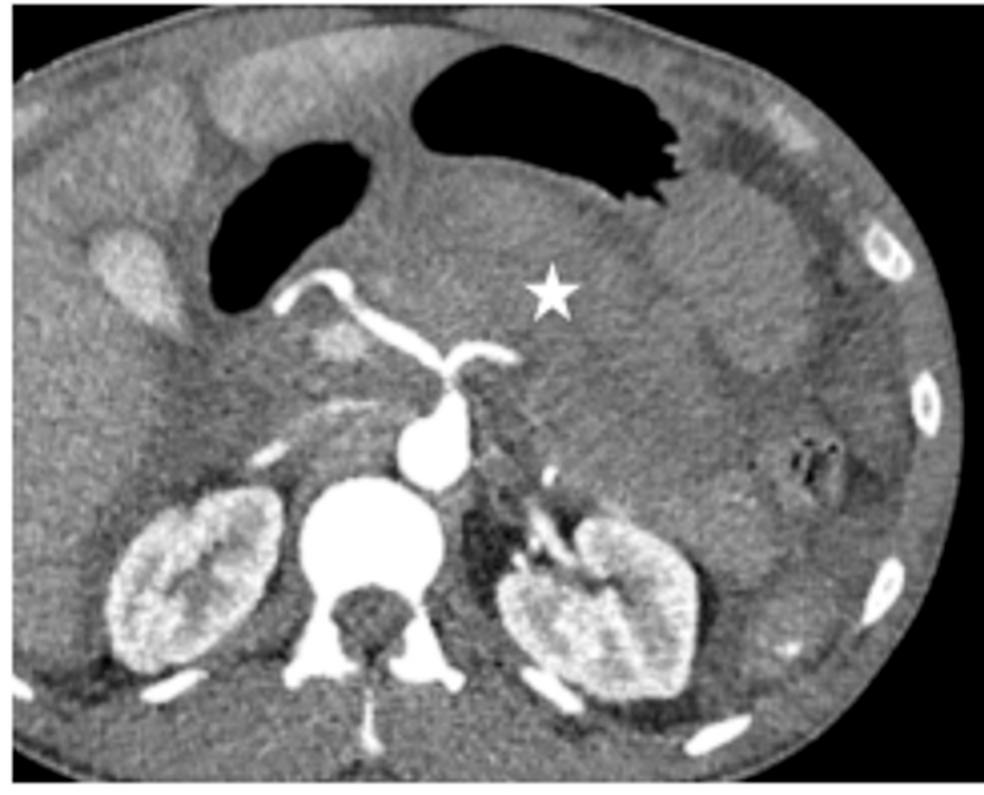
CT abdomen demonstrating a fluid collection in the lesser sac representing hemorrhage
